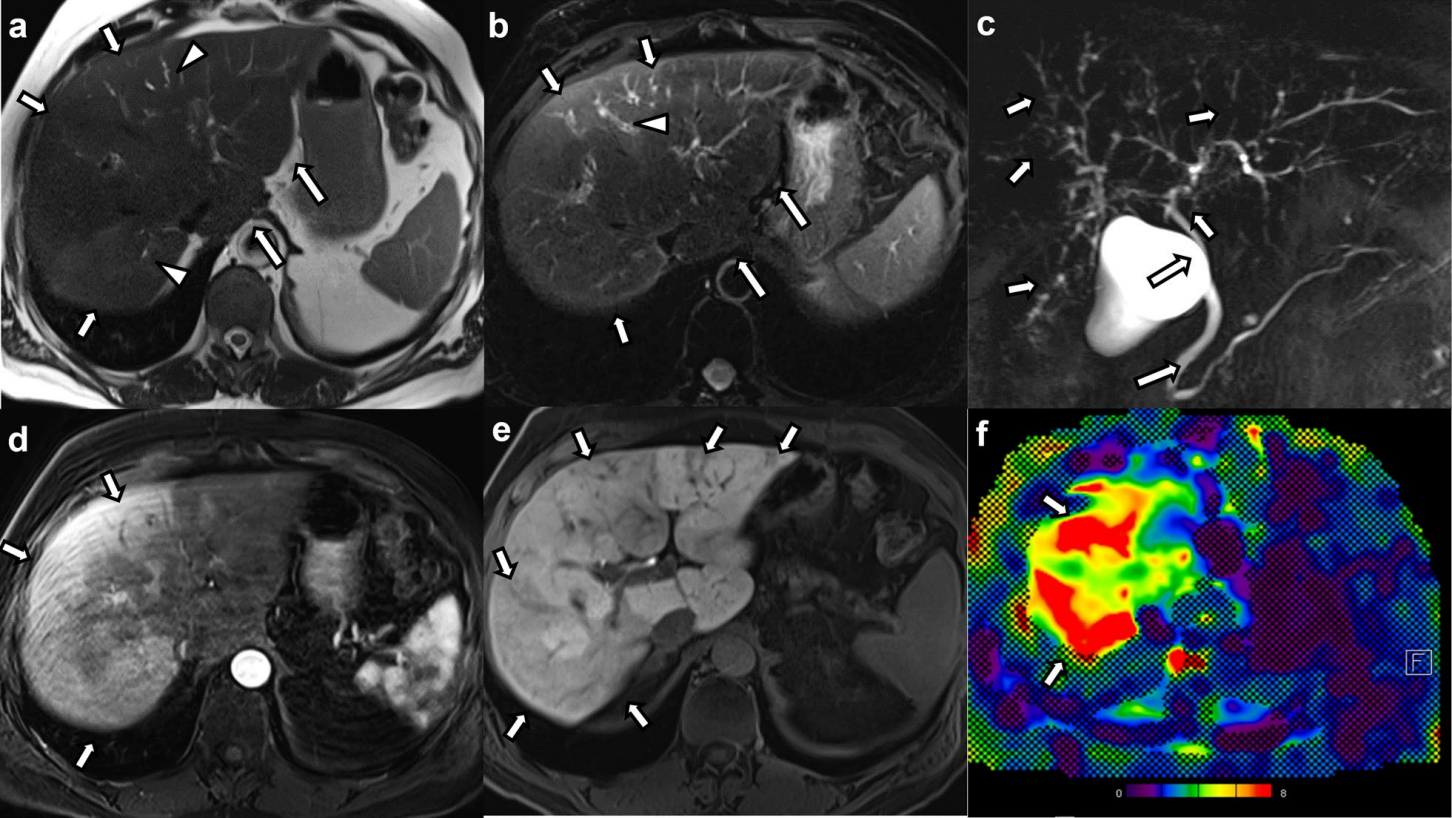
61-year-old male patient with COVID-19-associated SSC after severe COVID-19 infection and prolonged ICU stay. a Axial non-fat-suppressed and (b) fat-suppressed T2-weighted images show mild hyperintense signal changes predominantly in the right hepatic lobe (arrows in a and b) with subtle bile duct dilatation (arrowheads in a and b). The caudate lobe is enlarged, and the hepatic contours are rounded (long arrows in a and b). c Maximum intensity projection MRCP image shows irregularities of the intrahepatic bile ducts with beading and multifocal structuring (arrows). The extrahepatic biliary tree is spared (long arrows). d Post-contrast images in the arterial phase show diffuse areas of patchy hyperenhancement especially in peripheral areas of the right hepatic lobe (arrows). d The post-contrast images in the hepatobiliary phase show patchy hypointense changes representing areas of decreased of hepatobiliary contrast agent uptake (arrows). f Color stiffness map from MR elastography shows increased stiffness of the liver parenchyma (arrows) as represented by the yellow to red color coding (mean liver stiffness was 6.4 kPa)
